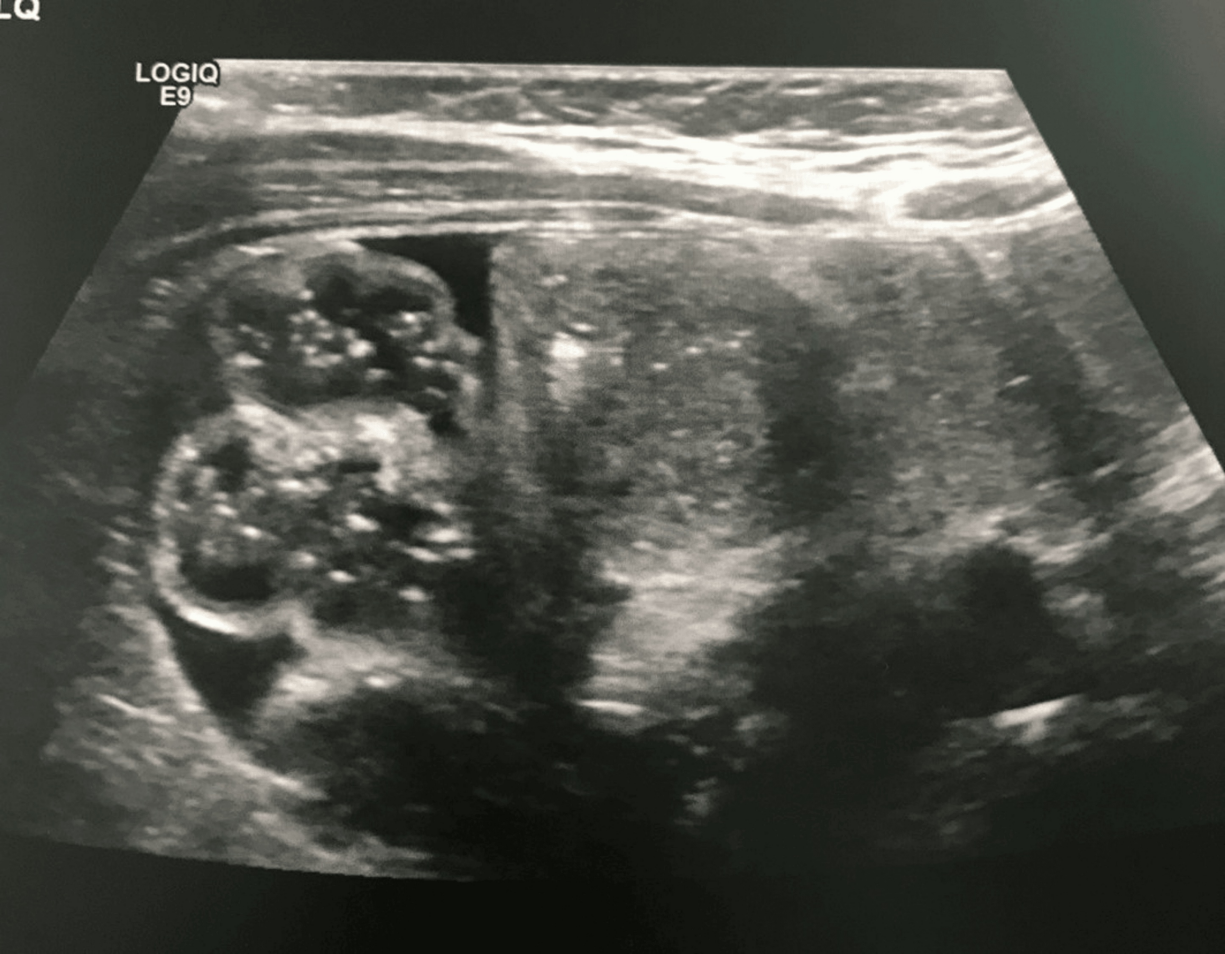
Day 1 abdominal ultrasound shows dilated bowel loops, and no masses are seen.
